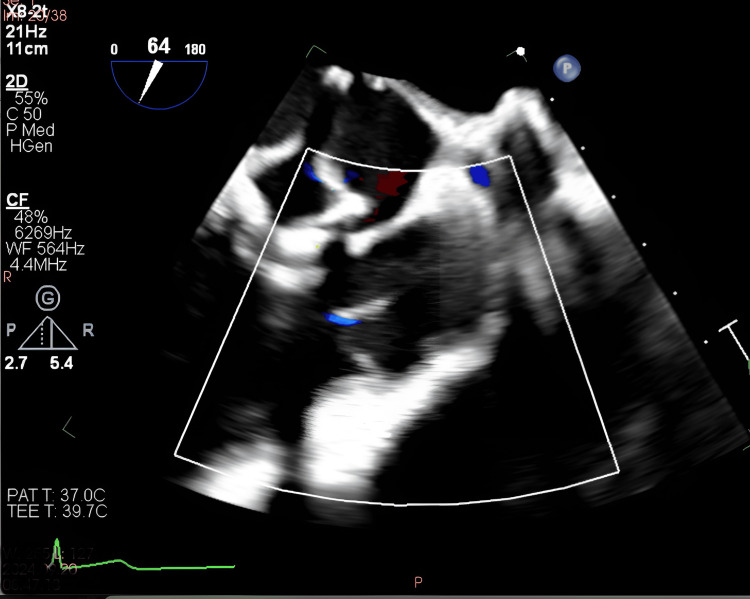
TEE showing pulmonic valve vegetation. TEE, transesophageal echocardiography
